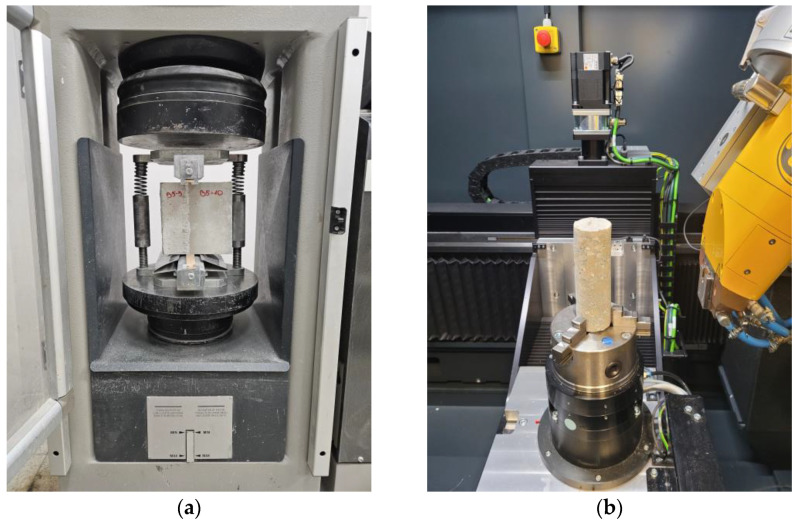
Performance of tests: (a) interlayer bond strength; (b) X-ray computed tomography.
